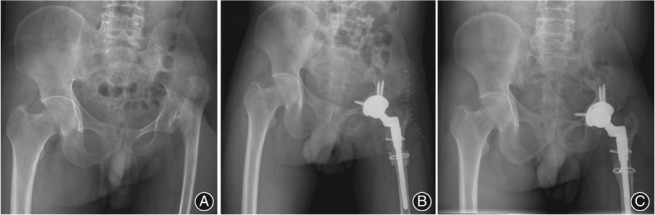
A 44‐year‐old man with Crowe type IV high hip dislocation secondary to pyogenic arthritis who had a quiescent period of 27 years between infection and right total hip arthroscopy (THA). (A) Preoperative anteroposterior (AP) pelvic radiograph. (B) Postoperative oblique hip radiographs immediately after right THA. (C) Postoperative AP pelvic radiograph 8 years after right THA.
